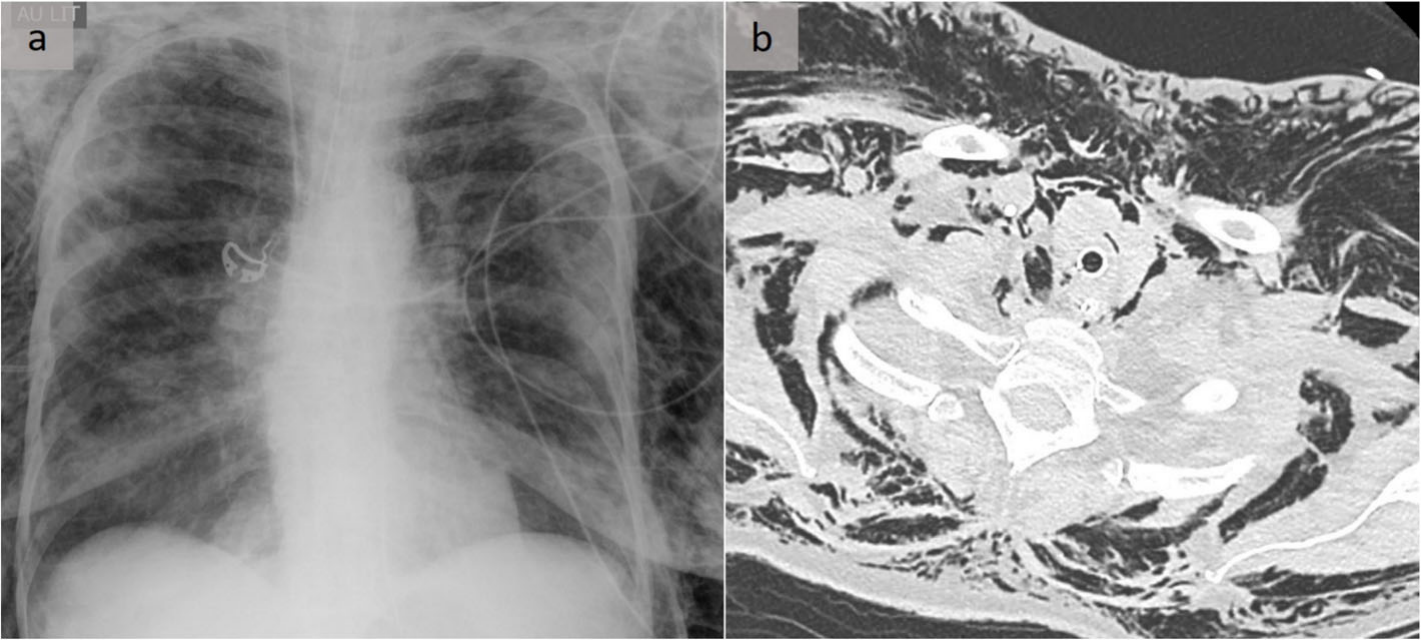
Pneumomediastinum related to tracheo-esophageal injuries. A patient resuscitated after cardiac arrest with pneumomediastinum related to both tracheal and esophageal injuries. The major finding on the chest X-ray (a) was the subcutaneous emphysema. Conversely, the pneumomediastinum is perfectly demonstrated on the axial CT scan (b)
